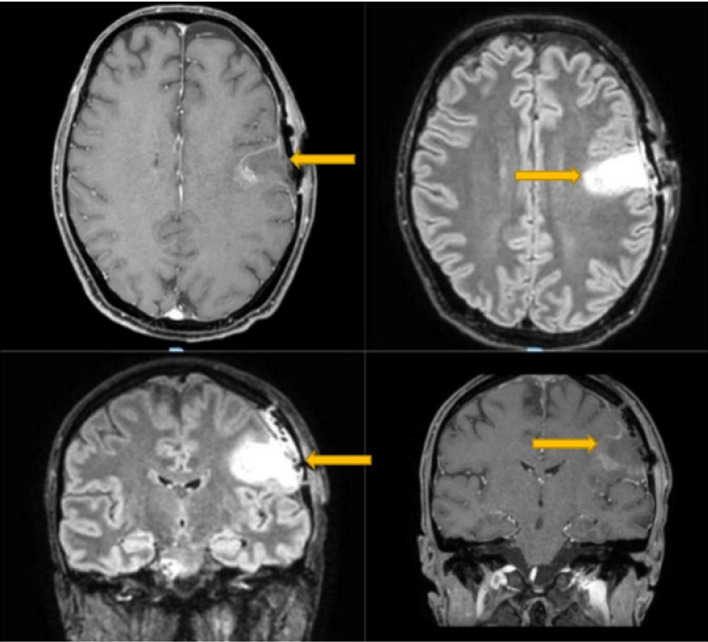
The postoperative magnetic resonance imaging did not show any new onset lesions near the surgical site or in the right operculum or in the subcortical fibers.
